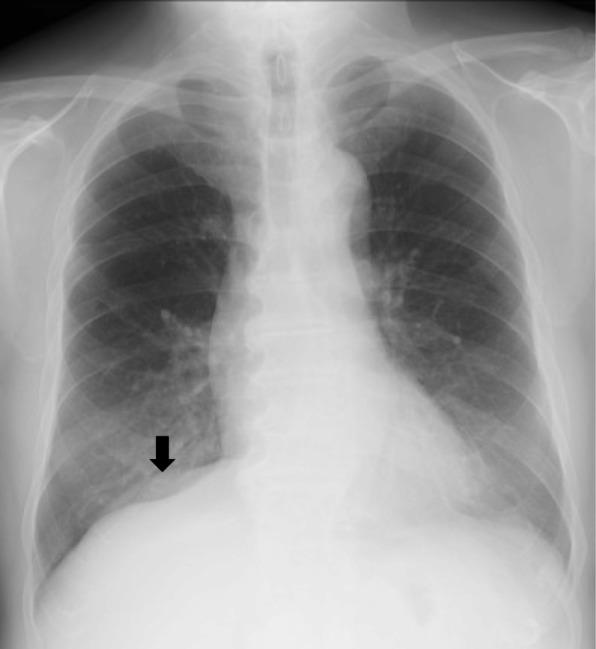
A chest X-ray film showing a double line on the right diaphragm (arrow)
